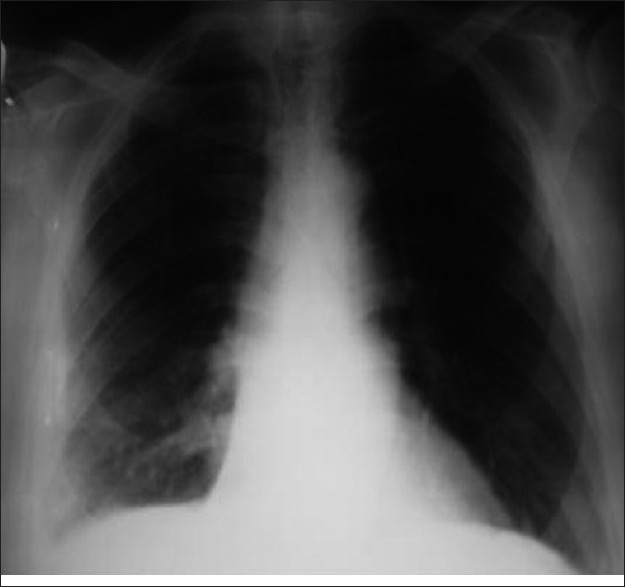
Posteroanterior chest X-ray after the cessation of cilazapril. The effusion has disappeared after the cessation of cilazapril
